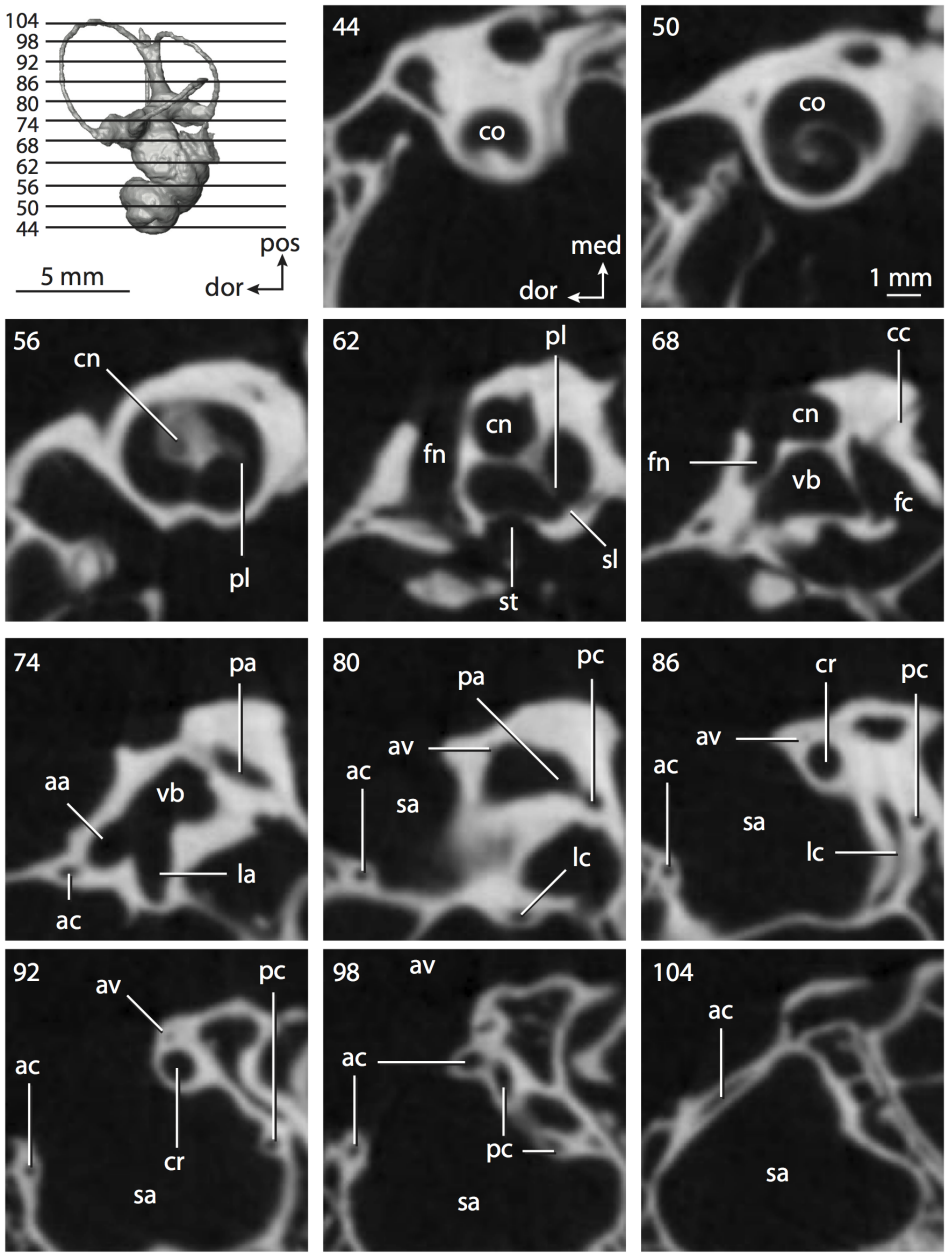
CT slices through ear region of*Lepus californicus*. Abbreviations listed at the end of the Materials and Methods section.
